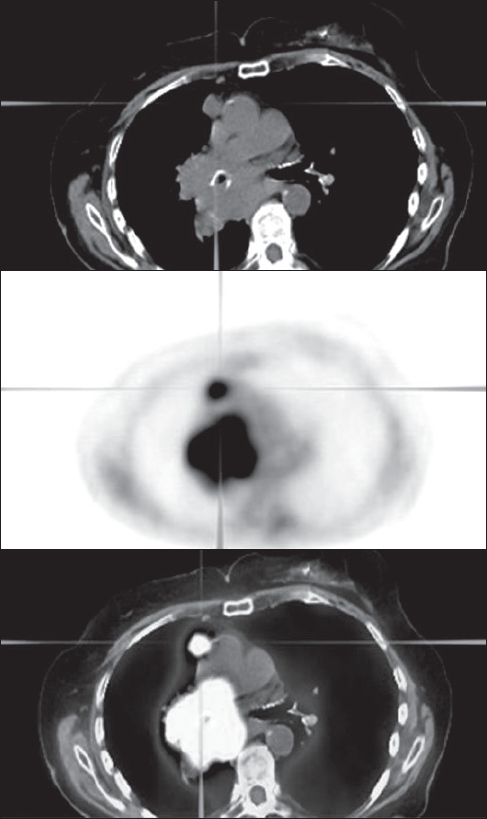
Non-small cell lung cancer. PET/CT images show a mass involving the right hilum with extension into mediastinum without extension to the contralateral mediastinum but a separable focus in the right superior mediastinum (stage IIIA) (Reprinted with permission of reference).[39]
